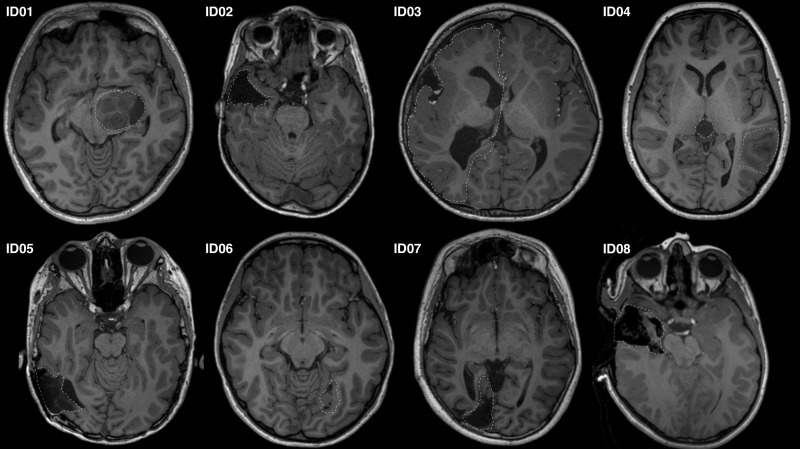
Representative axial T1-MRI images of all study participants. Images shown in radiologic convention. White dashed lines delineate the pathology/lesion or region of anatomical distortion involving the optic radiation.
